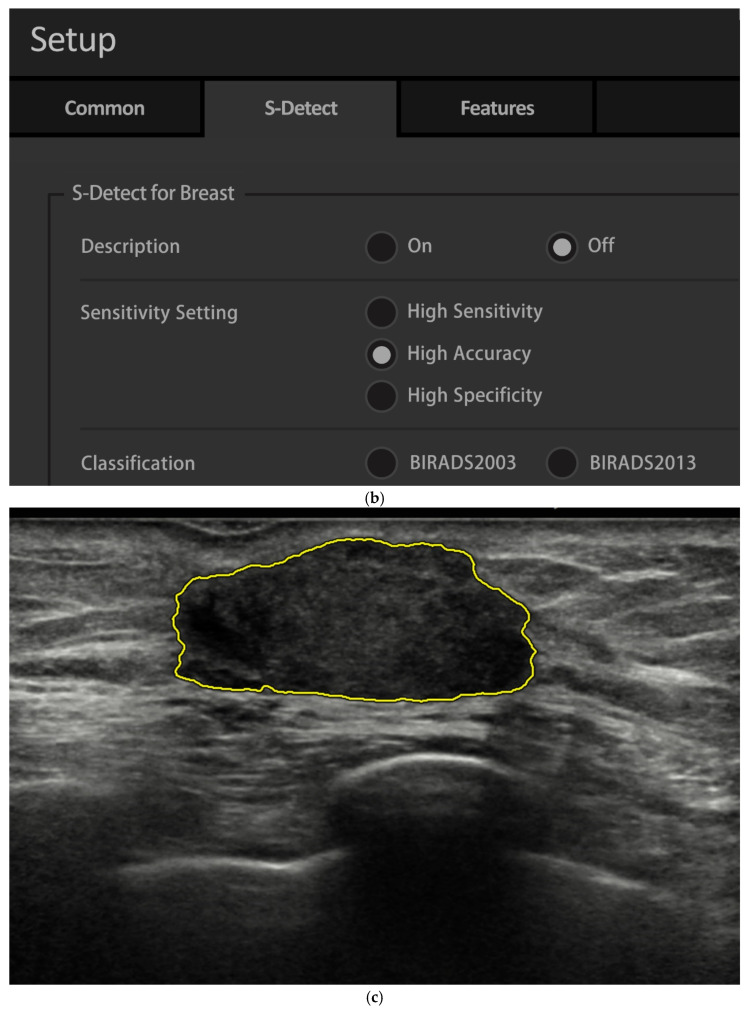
A 61-year-old woman diagnosed with invasive breast cancer. (a) A greyscale breast ultrasound serving as an example of how AI software (V2) can evaluate a suspicious lesion through three AI modes. (b) The S-detect system on the ultrasound machine provides three distinct AI modes: high-sensitivity (HSe), high-accuracy (HAc), and high-specificity (HSp). (c) Utilizing AI capabilities, the lesion of interest is automatically outlined when a sonographer places a marker within the targeted area.
